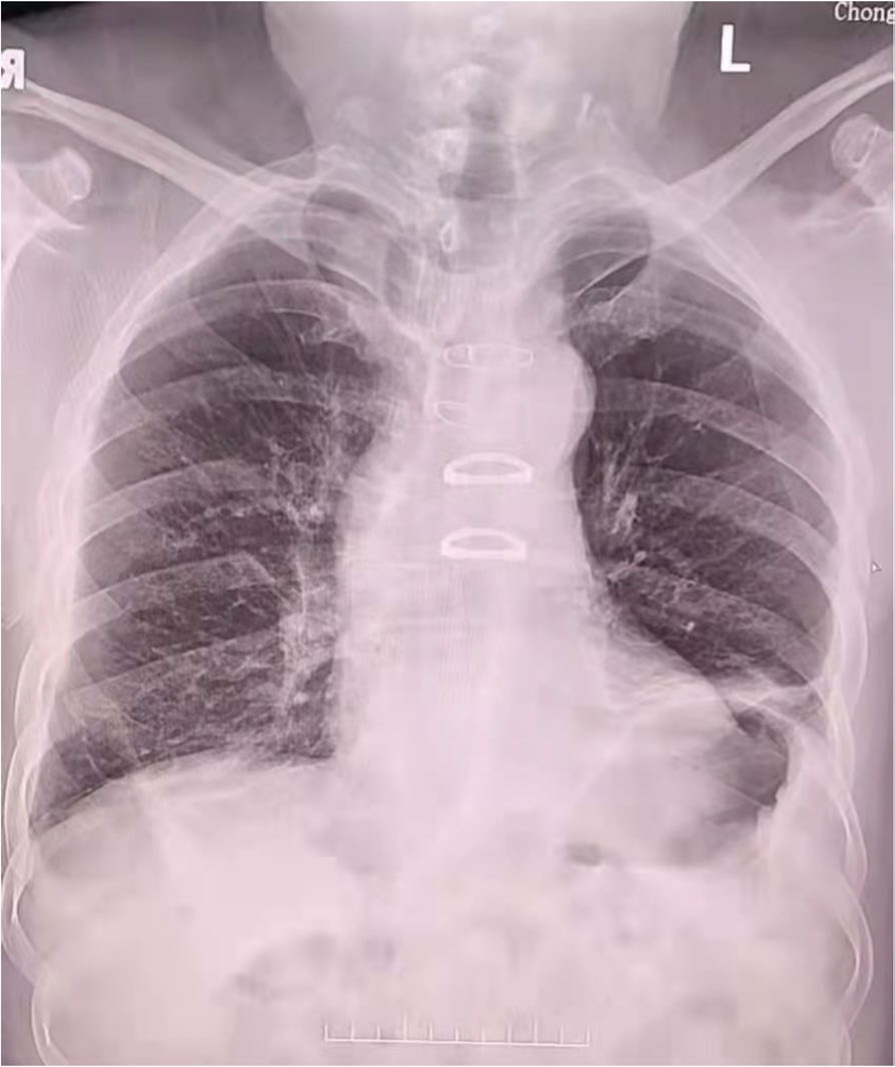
Chest x-ray performed at seven months after the start of antimicrobial treatment (March, 2022) showed the improvement of thoracic empyema
